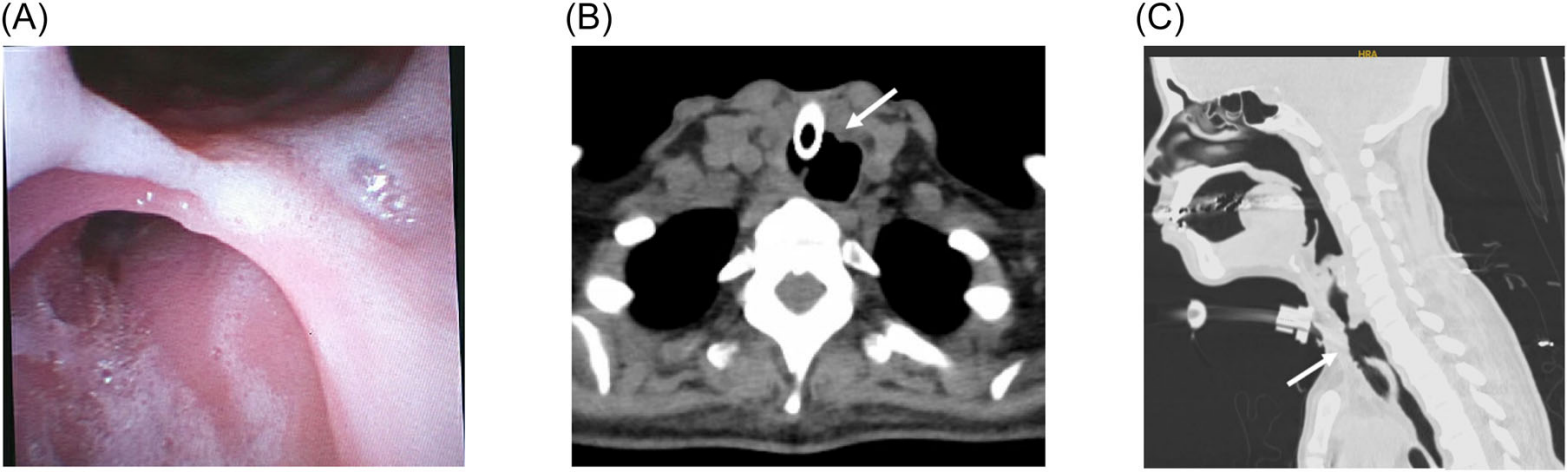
(A) Tracheoesophageal fistulae (TEF) visualized via bronchoscopy. (B, C) Axial and sagittal view of TEF on Computed tomography.
